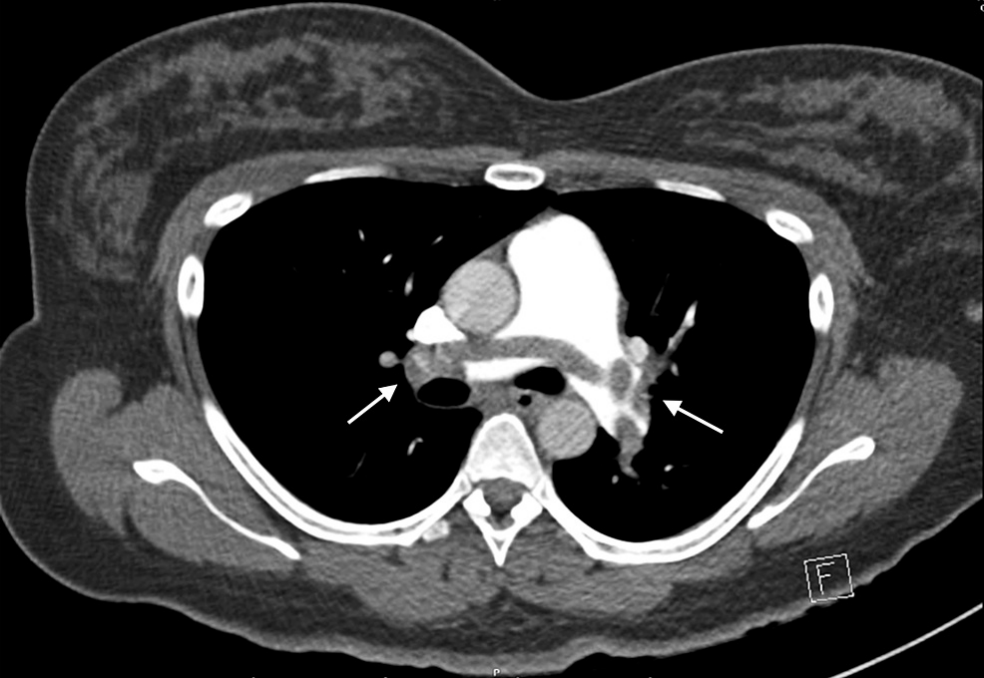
Axial images of the CTA of the chest with saddle pulmonary embolus (arrows)
